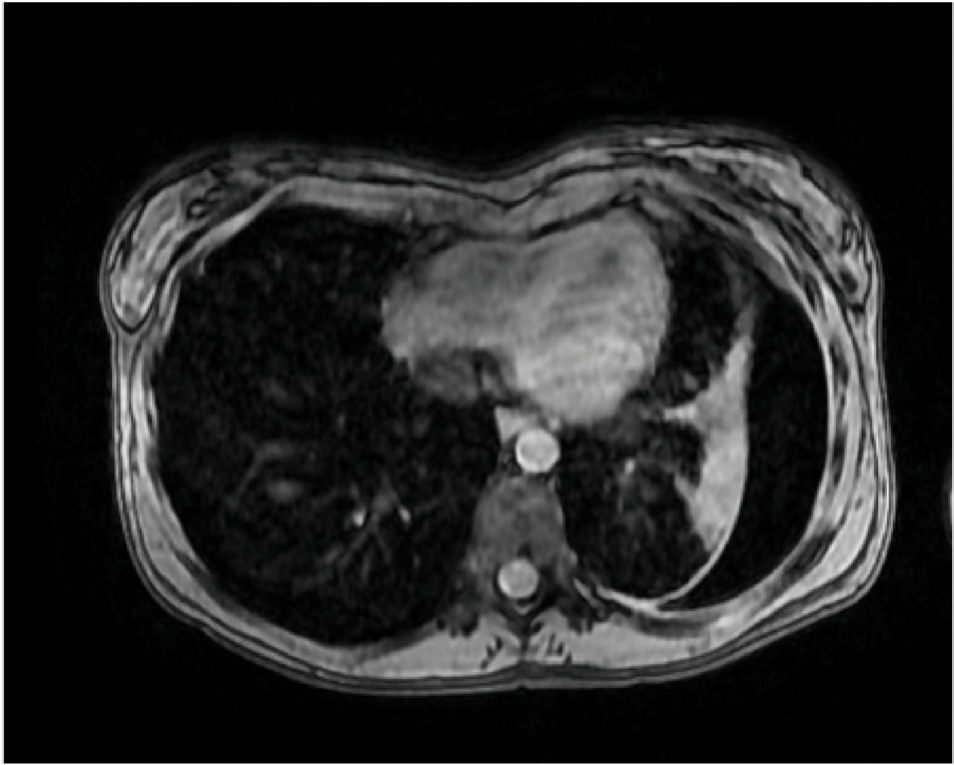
MRI of a female patient with tuberous sclerosis complex and lymphangioleiomyomatosis. Pneumothorax, which is a complication of lymphangioleiomyomatosis, with ipsilateral pulmonary atelectasis is noted.
